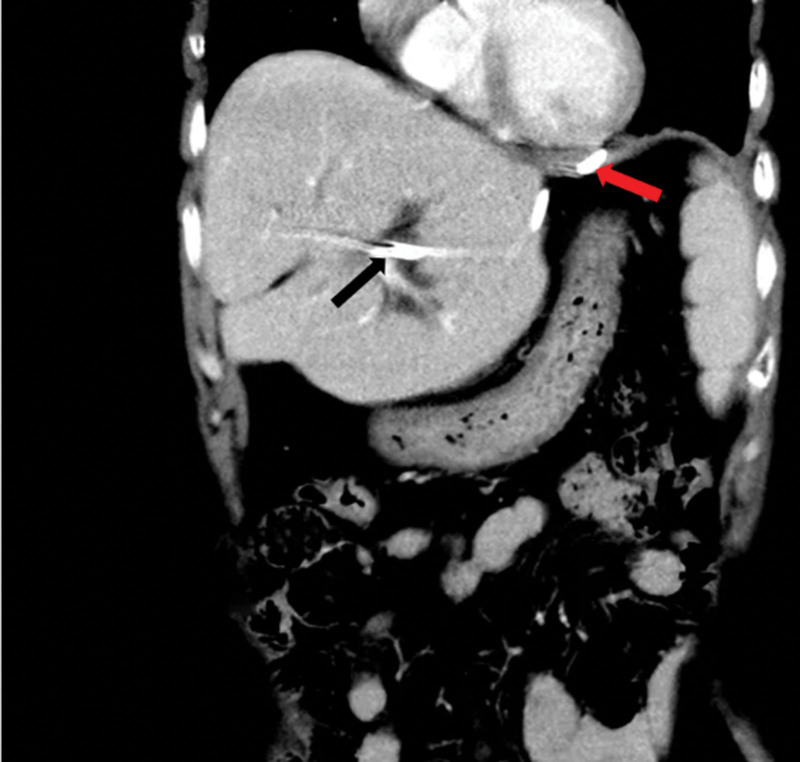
CT image scans showing 2 drainage tubes were successfully inserted into the dilated bile duct (Black arrow) and subphrenic abscess (red arrow) and adequate drainage. CT = computed tomography.
